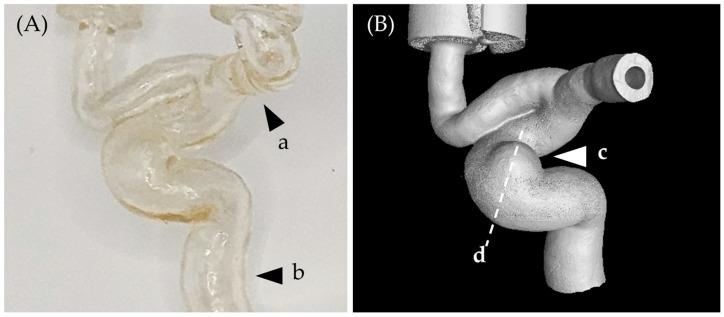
A 3D-printed ICA aneurysm model. (A) Optical image (a: distal end, b: proximal end), (B) micro CT image (c: ICA aneurysm, d: cross section location).
